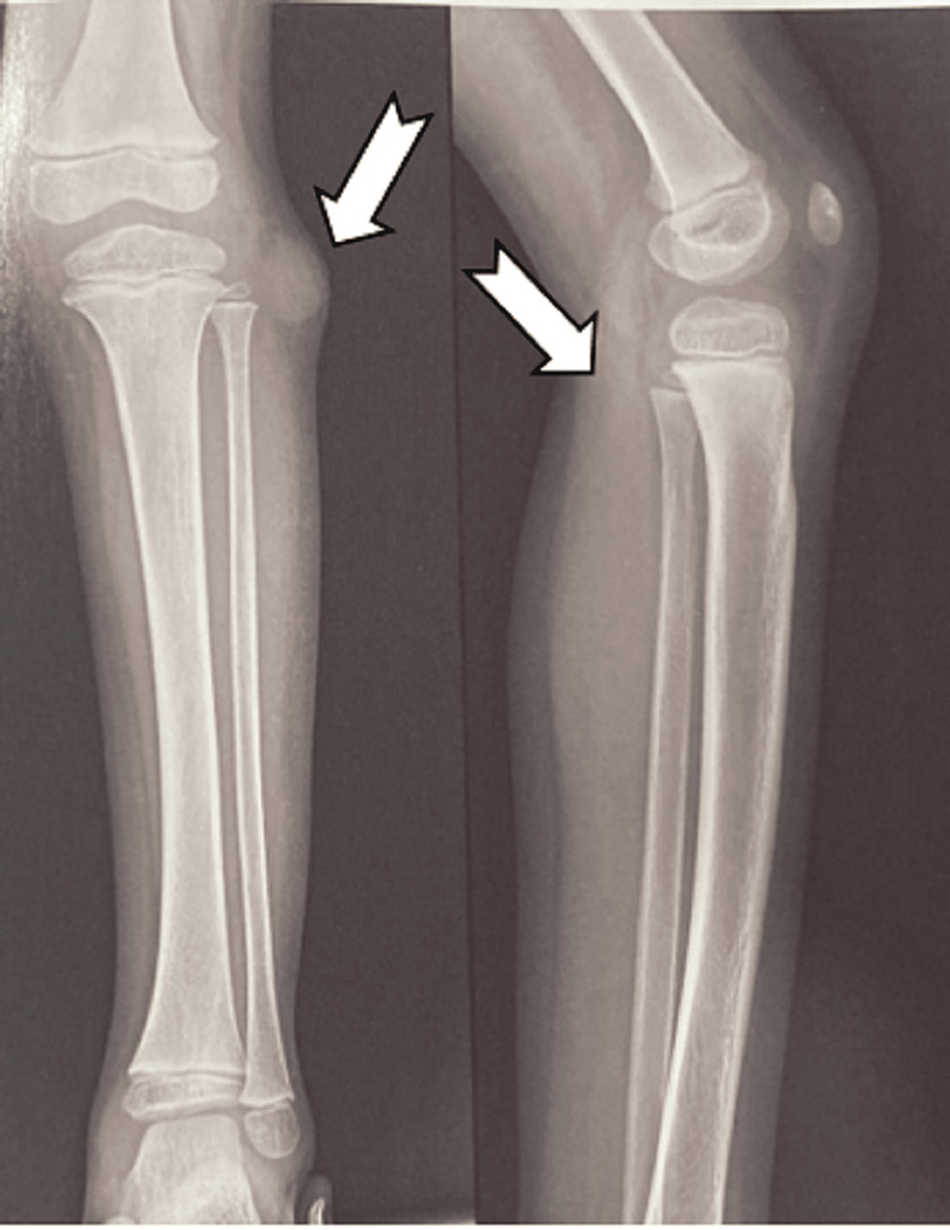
X-ray of left knee with leg anteroposterior and lateral view showing soft tissue swelling at the lateral aspect of the proximal leg near the head of the fibula
